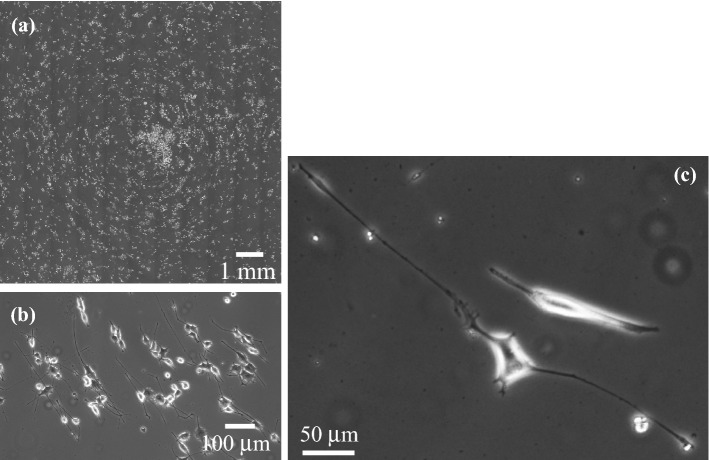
Phase-contrast images of PC12 cells at *t* = 72 h (92 h after adding the NGF) under 78 kHz ultrasonication with the transducer input voltage of 30 V_pp_.
